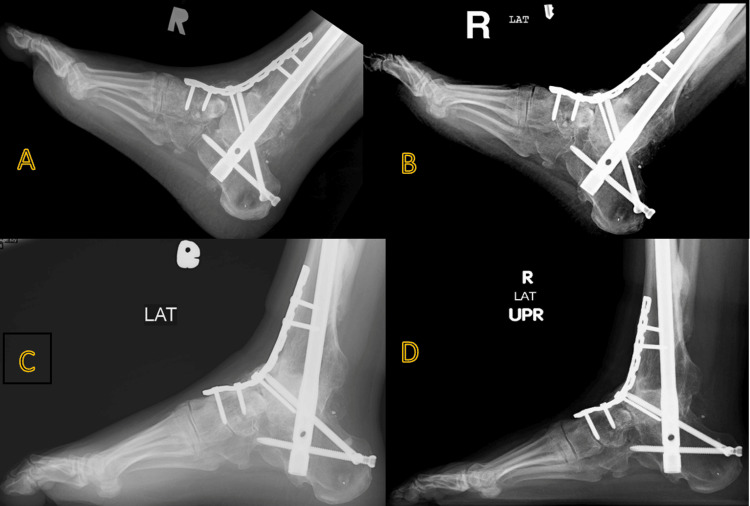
Right lateral foot X-ray (A) Two months post-procedure; (B) One year post-procedure; (C) Two years post-procedure; (D) Three years post-procedure
